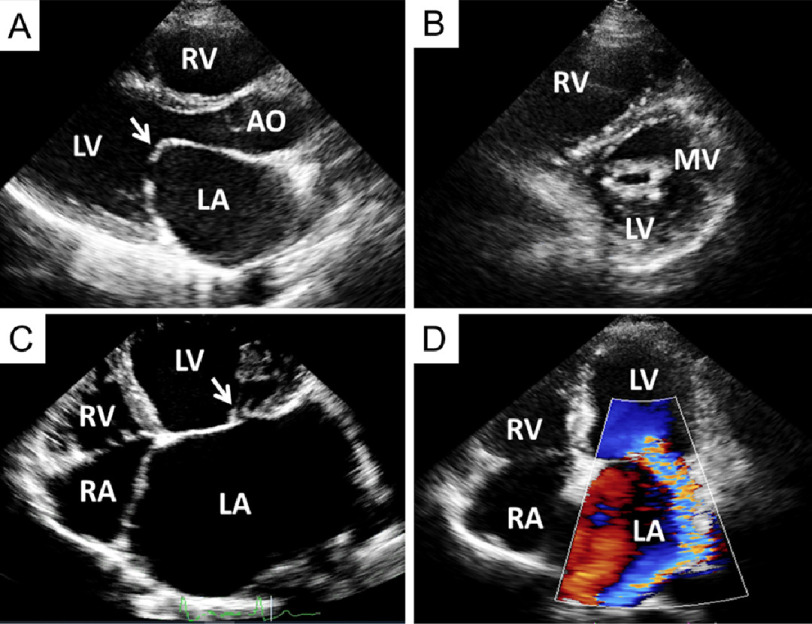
Transthoracic echocardiography from a patient with severe mitral stenosis in parasternal long-axis (A) and short-axis (B) views. Both leaflets are thickened with a pliable anterior leaflet on the long-axis view and fusion of both commissures on the short-axis view. The anterior leaflet has been described as opening in a “hockey stick” appearance in parasternal long axis view (arrow). Transthoracic echocardiography from a patient with severe mitral regurgitation, apical four-chamber view. A coaptation defect of mitral valve can be detected (arrow) with severely dilated left atrium (C). Color flow mapping showing severe mitral regurgitation (D). LA = left atrium; LV = left ventricle; Ao = aorta; RV = right ventricle MV = mitral valve area, RA = right atrium.
